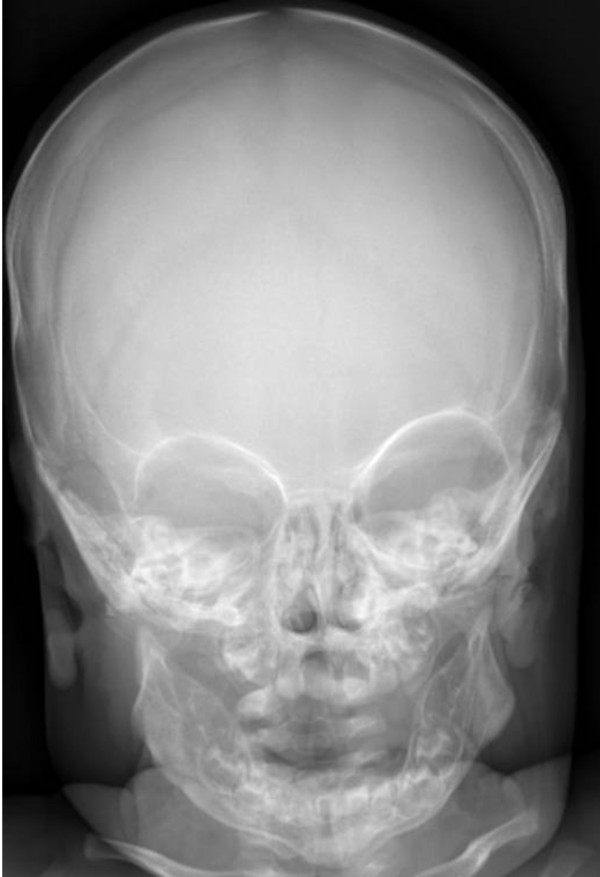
Anteroposterior radiograph of the skull showed massive sclerosis of the skull bone associated with significant cortical hyperostosis and enlargement of the mandible secondary to cortical new bone formation.
